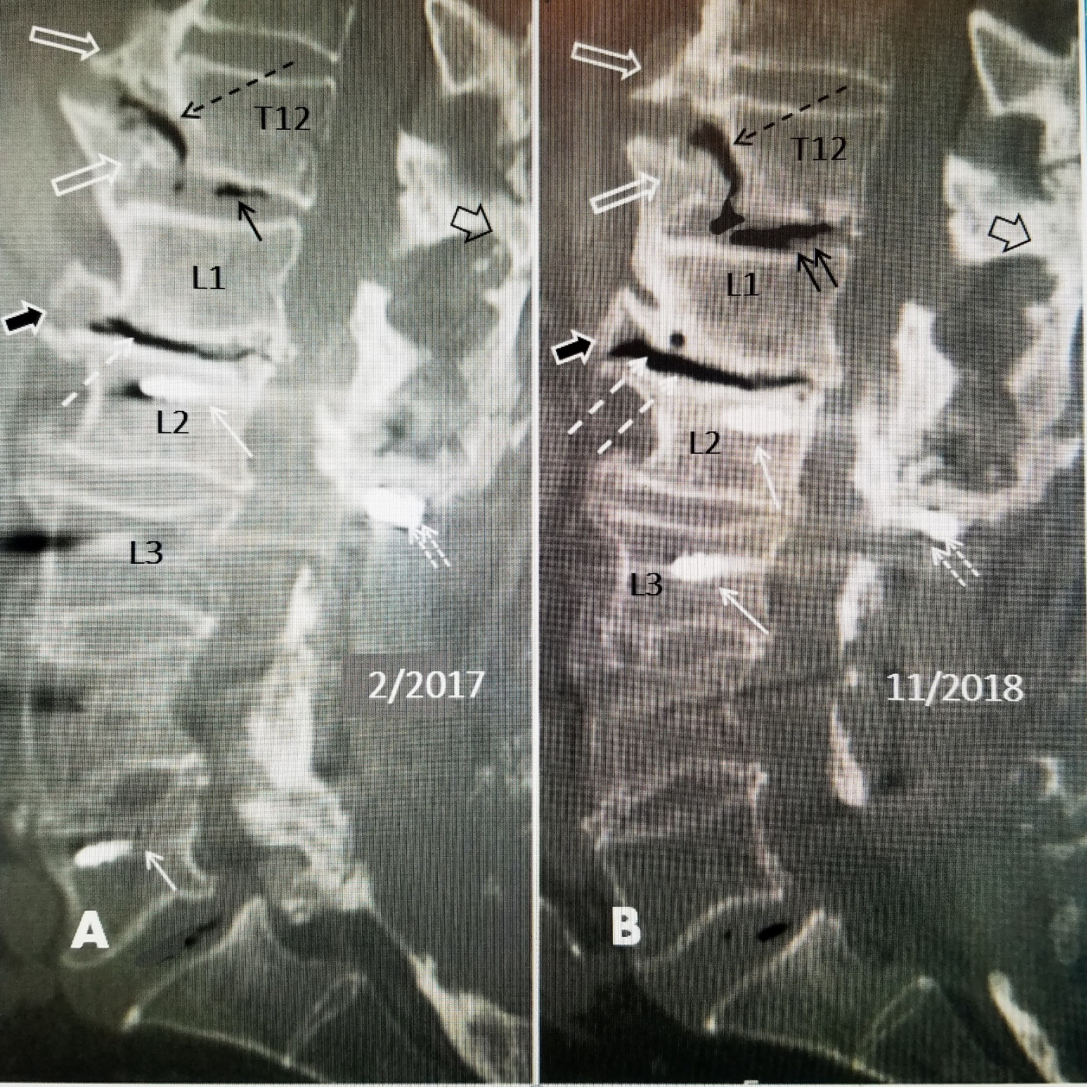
Lumbar and lower thoracic sagittal computerized tomography (CT) scan from February 2017 and November 2018 showing increasing vacuum changes at T12-L1 extending into the vertical T12 fracture A: Sagittal CT scan from February 2017 showing an anterior vertical avulsion fracture of inferior endplate of T12 (open white arrow) associated with vacuum signal change within the fracture (dashed black arrow). The T12-L1 disc space has vacuum changes (solid black arrow). There is also an osteophyte at L1-L2 ventral to the disc space (solid black arrow with white border). Vacuum change is also seen within the L1-L2 disc space (dashed white arrow). The supraspinous ligaments are calcified (open black arrow). Parts of pedicle screws are identified in the vertebral body (solid white arrows). B: Sagittal CT scan from November 2018, 21 months later, showing worsening vacuum changes compared to 2017. The avulsion fracture of the inferior endplate of T12 is unchanged in position (open white arrow) however the vacuum changes along the fracture line are more extensive (dashed black arrow). The osteophyte at L1-L2 (solid black arrow with white border) is unchanged. The vacuum change at T12-L1 disc space is more extensive (two small black arrows) as well as within the L1-L2 interspace (two dashed white arrows). The pedicle screws are marked at L1 (solid white arrow) and into the posterior bone (two small dashed white arrows). There is solid bone growth bridging the supraspinous ligament (open white arrow with black border) contributing to the spinal rigidity.
